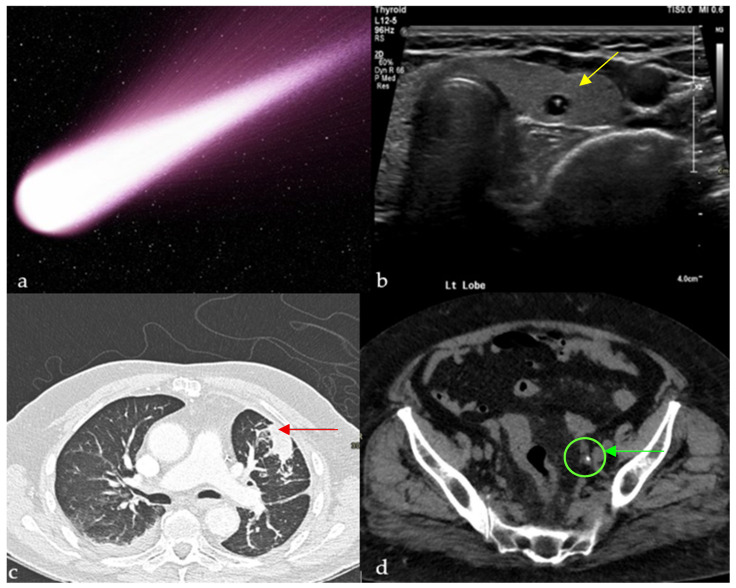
Small calcific/crystalline highly reflective structure causing reverberation artifact on ultrasound of the thyroid (yellow arrows) (b), axial CT of the chest showing a curvilinear opacity extending from a subpleural mass toward the ipsilateral hilum with distortion of vessels and bronchi (red arrow) (c), and tail of soft tissue extending from a calcification, representing the collapsed/scarred/thrombosed parent vein on a non-enhanced CT of the pelvis (green arrow) (d), all resembling a comet tail (a).
